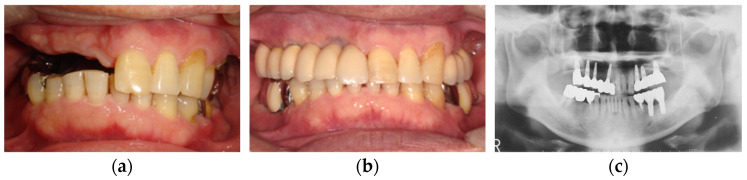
Implant-supported prostheses for the patients with anterior hyperfunction: (a) pre-treatment; (b) post-treatment; (c) a panoramic X-ray image of post-treatment. Source: the authors.
